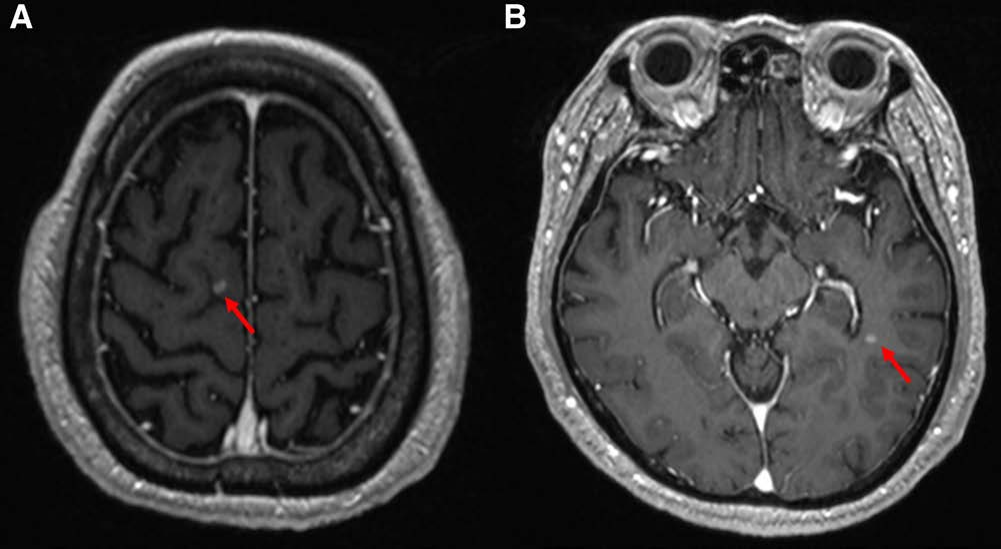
Brain MRI of the patient. T1-weighted (MPRAGE sequence showed multiple enhanced tumors at multiple sites. Arrows: Metastatic lesions in the right frontal and left temporal area. MPRAGE = magnetization prepared rapid gradient echo, MRI = magnetic resonance imaging.
